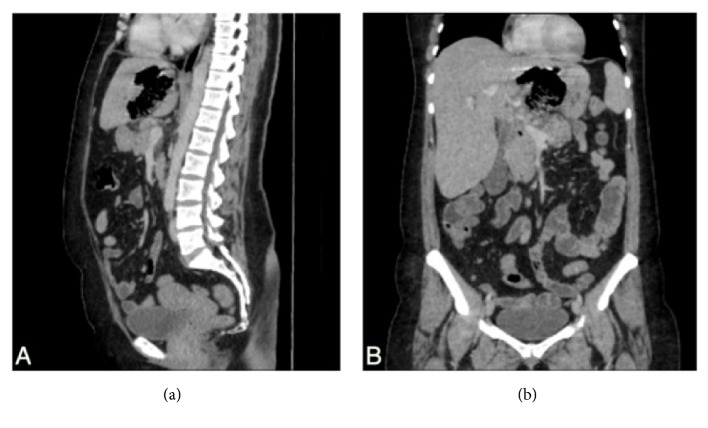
Sagittal (a) and coronal (b) abdominal CT scan with oral and IV contrast. Mixed collection containing air and debris measuring 8x7x5.5 cm is present. The lesion is spreading at the posterior aspect of the left lobe at segments II and III. Emphysematous changes of the adjacent parenchyma are noted.
